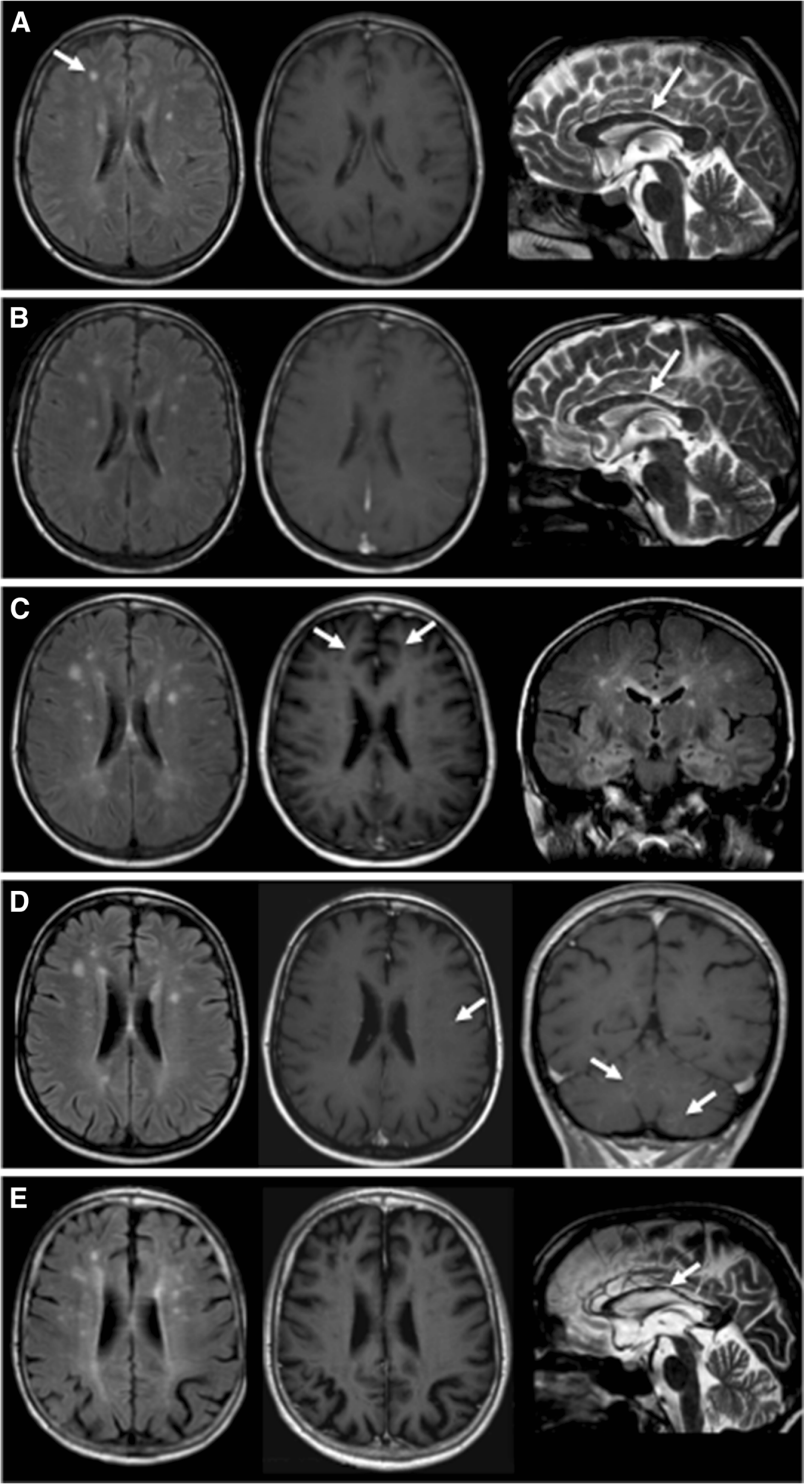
Brain magnetic resonance imaging: axial FLAIR (first column), axial Gd -T1 (second column), sagittal T2 (third column: A, B, E), coronal FLAIR (C) or coronal Gd-T1 (D). A. March 14th, 2008 (first relapse): T2/FLAIR hyperintense non-enhancing lesions of centra semiovalia (arrow) and corpus callosum (arrow) white matter. B. April 1st, 2008 (first relapse, follow-up; diagnosis of SS): increased number and intensity of the T2/FLAIR hyperintense non-enhancing lesions of brain white matter, corpus callosum (arrow), also extending to cerebellum (not shown). C. May 15th, 2008 (third relapse): further increase of lesion number and intensity, some of the lesions are now enhancing (arrow). D. November 25th, 2008 (fourth relapse): stabilization of the supratentorial lesions, but new multiple contrast-enhancing brain (arrow) and cerebellar lesions (arrows). E. September 6th, 2012 (long-term follow-up): no evidence of disease activity with ensuing brain atrophy, as shown by dilatation of cortical sulci and loss of brain volumes, including at the corpus callosum (arrow).
